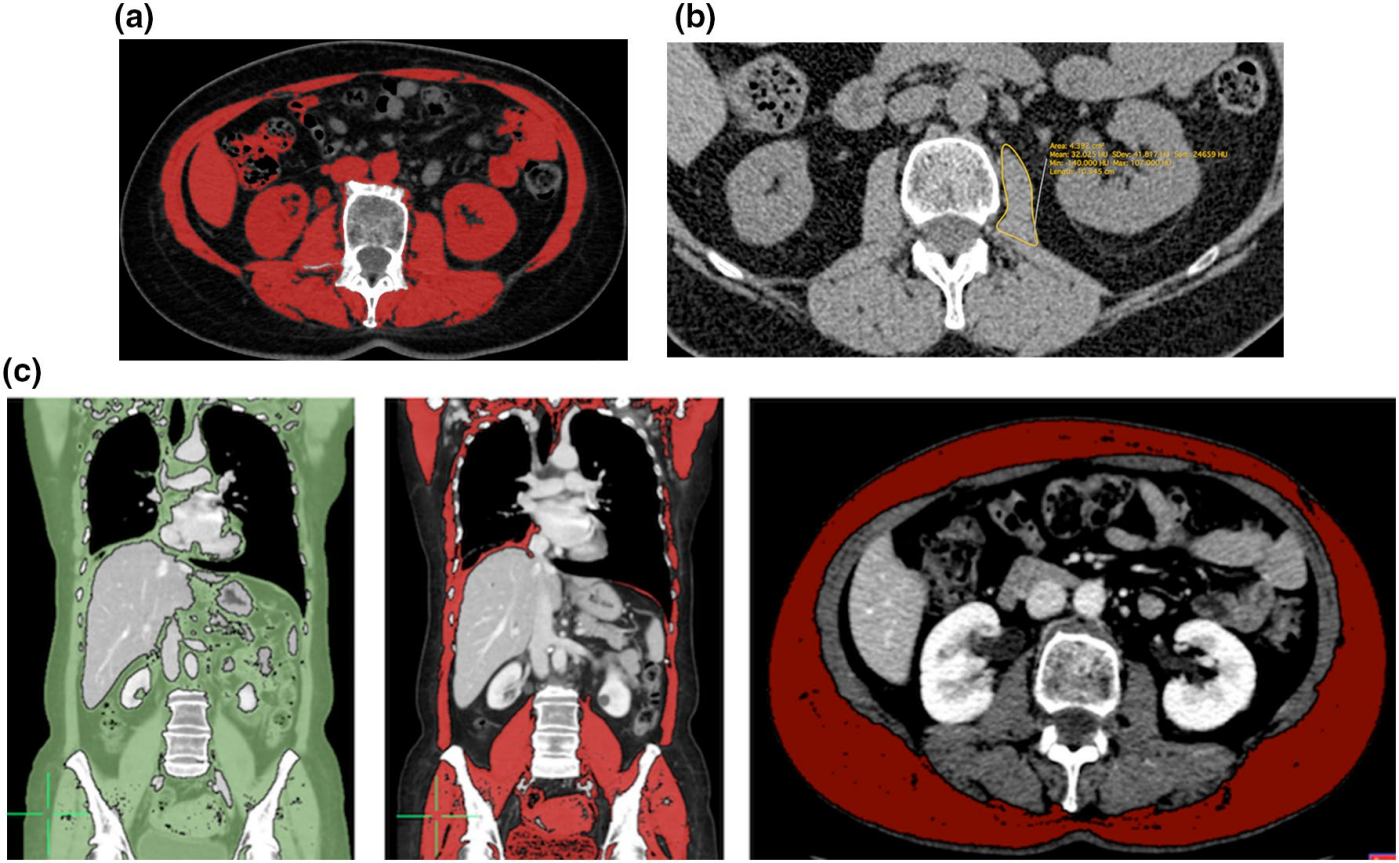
a Abdominal CT images at the third lumbar vertebra. Pitfalls are represented by incorrect segmentation of muscular tissue due to the fact that growing algorithms could be unable to distinguish muscular tissue from parts of the bowel and solid abdominal organs (kidney, major vessels and liver). Manual correction is essential. b Classical cross-sectional area evaluation at the L3 level showing muscle area and muscle density in Hounsfield unit. c Whole-body CT images taken with different planes showing some examples to estimate fatty tissue (green), muscular tissue (red), and subcutaneous fat (purple). Note that seeding method has been used; therefore, manual correction by an expert reader is mandatory
